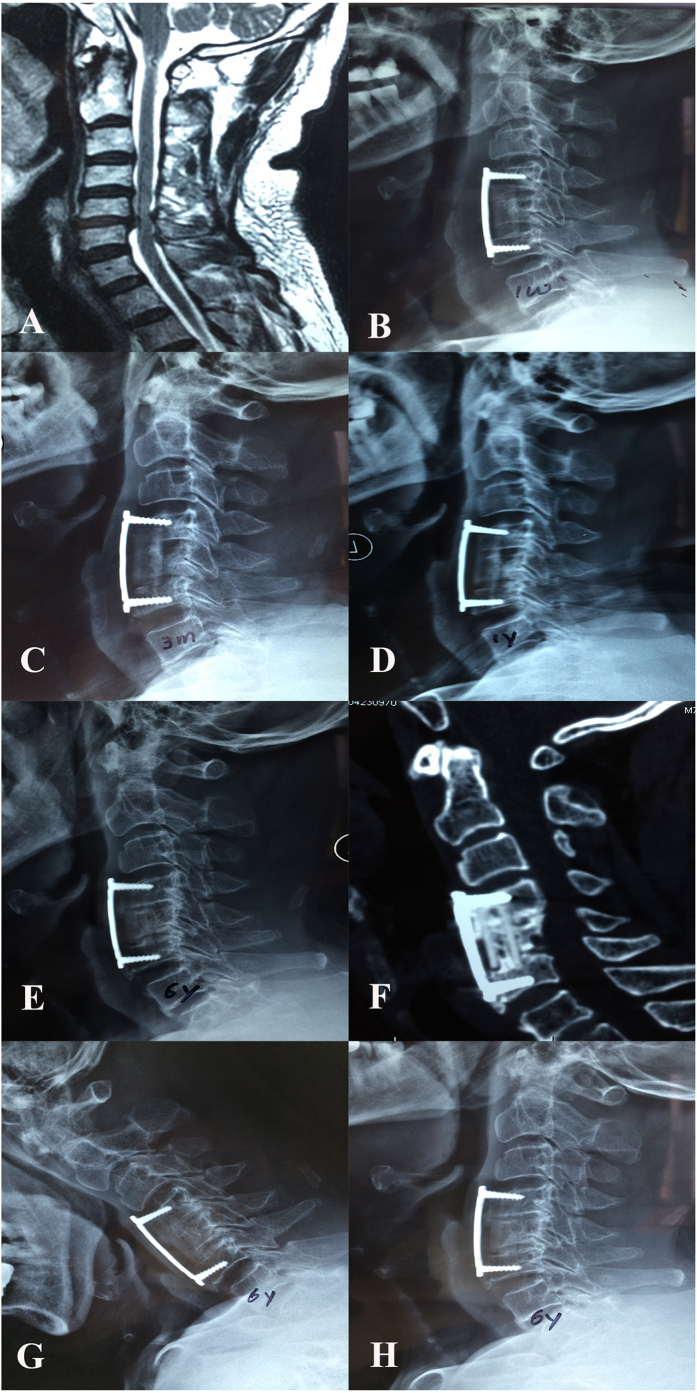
A 73-year old man who underwent 1-level ACCF with an n-HA/PA66 strut. Preoperative MRI (A) scans revealed the lesion segment. A cervical lateral X-ray at one week after surgery (B) revealed that the strut was in the appropriate position, but the anterior titanium plate was too long. The n-HA/PA66 strut sank into the upper endplate, and internal fixation was dislodged at 3 months after surgery (C). At the 1-year follow-up, the subsidence of the n-HA/PA66 strut and the internal fixation migration were aggravated (D). A postoperative lateral X-ray (E) and 3D-CT (F) at the 6-year follow-up revealed bony fusion between the autograft inside the strut and the adjacent vertebrae. Osteophytes were also observed at the gap between the anterior plate and the upper/lower vertebral bodies. The flexion (G) and extension (H) radiographs revealed solid fusion in the fusion segment.
